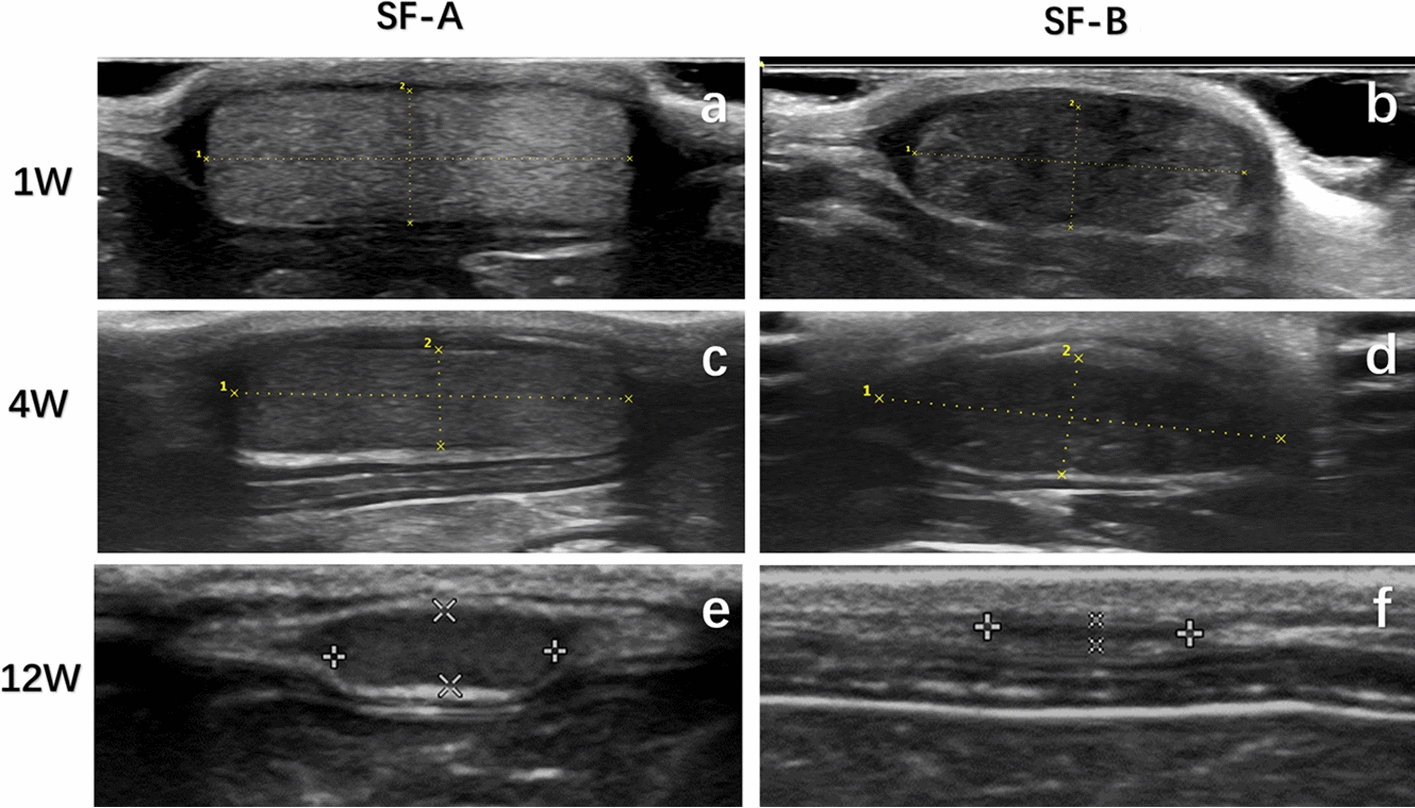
Examination of B-mode ultrasound of two types of silk fibroin scaffolds. B-mode ultrasound examination of silk fibroin scaffolds in SF-A group (a, c, e) and SF-B group (b, d, f) at 1, 4, and 12 weeks after implantation
